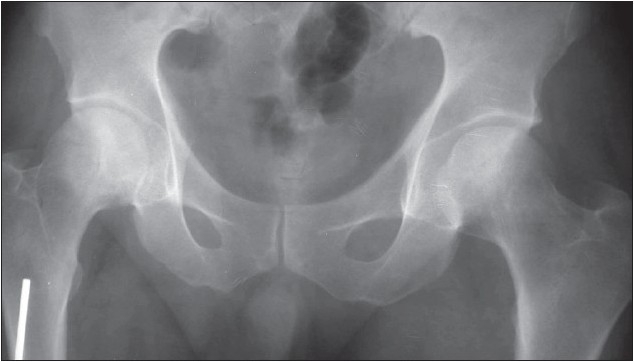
Anteroposterior radiograph of pelvis. No lucency, sclerosis or subchondral collapse noted in the femoral head. Joint space is preserved.
